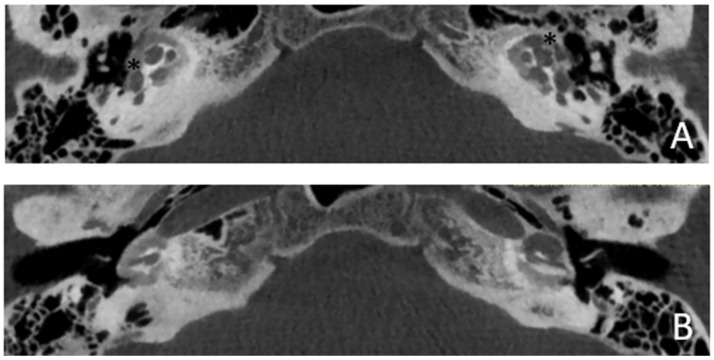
CT images of patient #4 with otosclerosis. (A,B), axial plane. The cavity around the cochlea is clearly visible as well as the communication of the cavitating osteorarefation with the cochlea, marked with asterisks.
